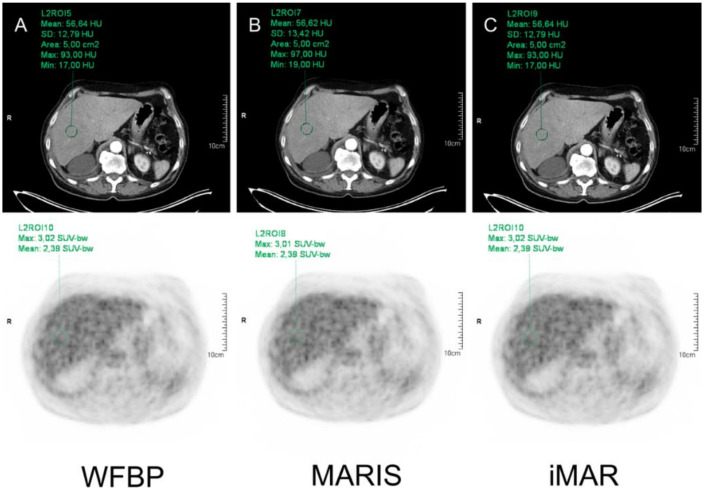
Example of HU and SUV measurement in the liver in WFBP (A), MARIS (B), and iMAR (C).
